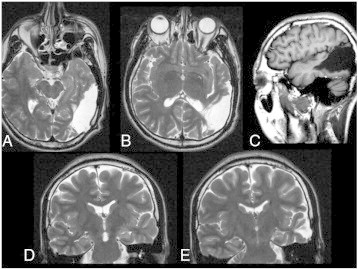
MRI shows a large porencephalic cyst in the left parietotemporal region with atrophic changes in the surrounding cortex (A, B; axial T2 weighted-imaging, C; sagittal T1-weighted imaging), but no atrophy or abnormal signal alteration is seen in the bilateral hippocampi (D, E; coronal T2-weighted imaging).
